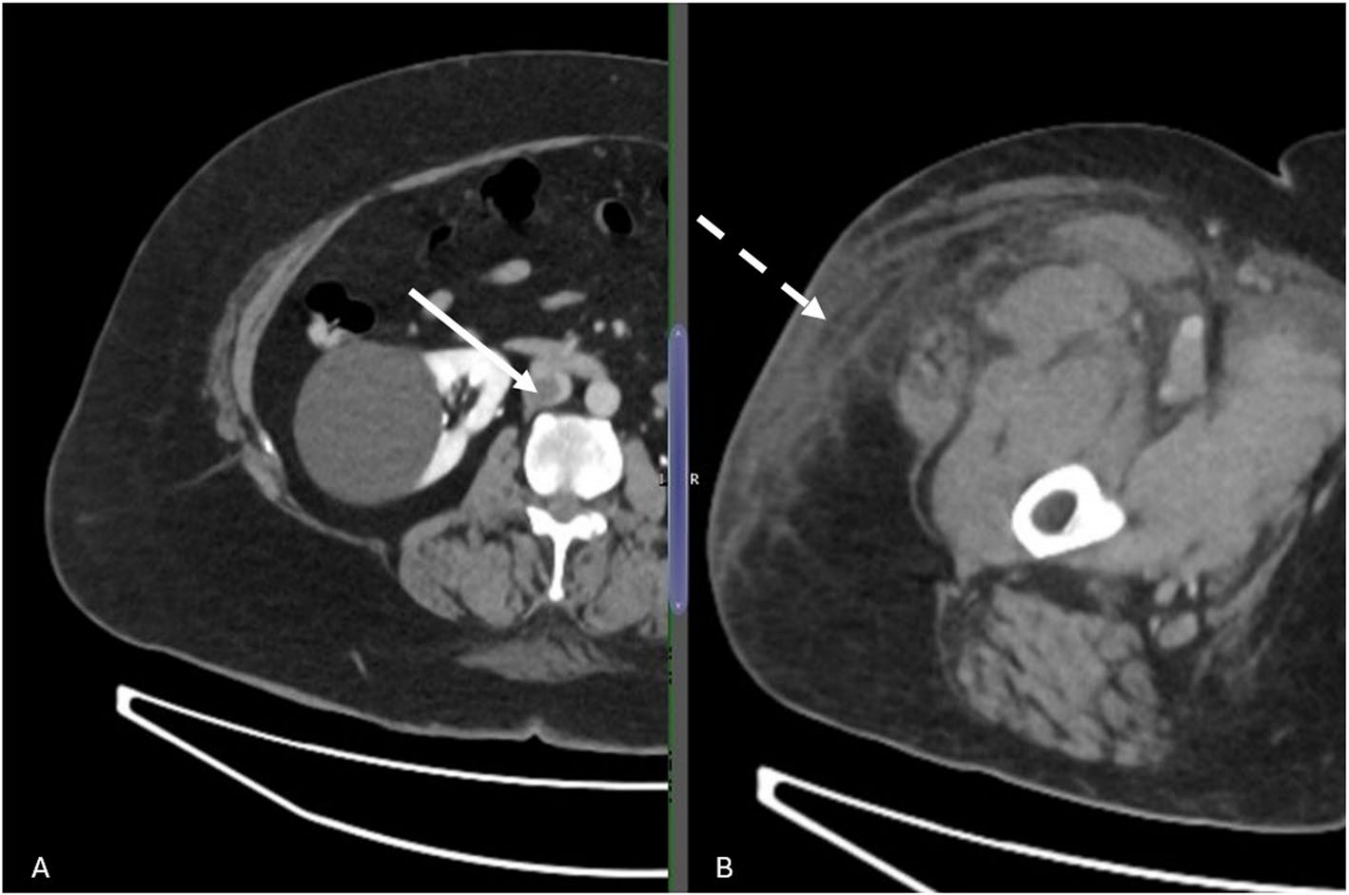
Computed Tomography imaging showing proximal extent of thrombus in the IVC (white arrow) a, and significant right lower limb superficial oedema (dashed arrow) b
